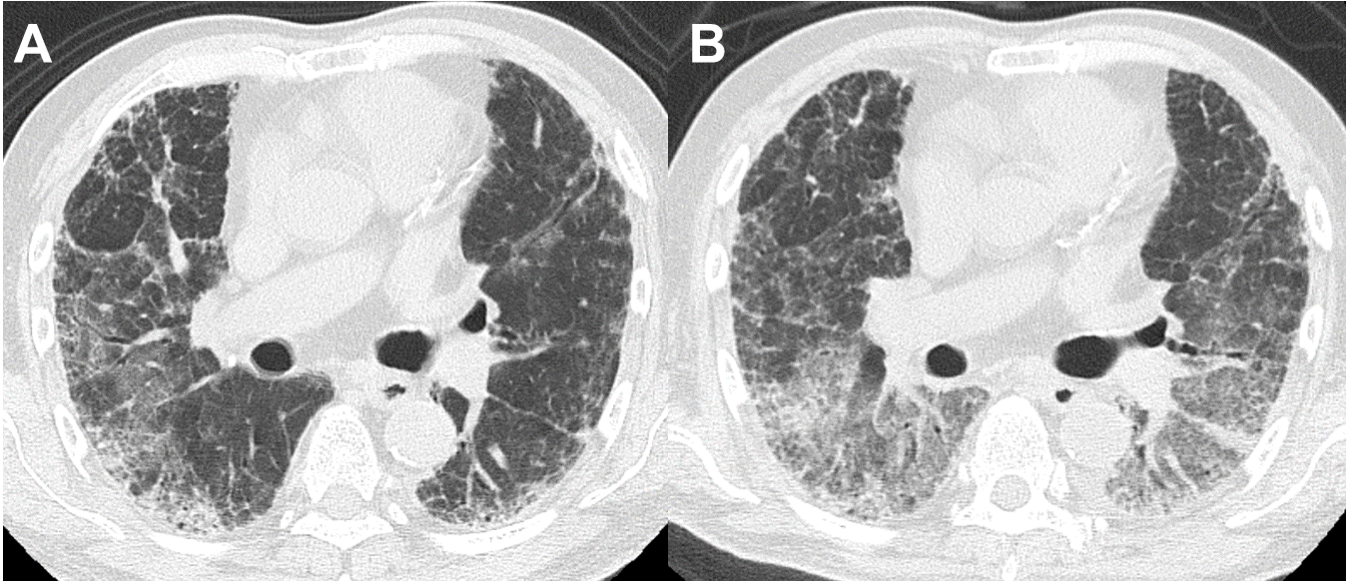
A patient in their 60s with chronic hypersensitivity pneumonia. (A) HRCT before the BAL procedure showed reticular shadows with bronchodilation in the peripheral lesion. (B) Two days after the BAL procedure, which was performed from B3b on the right lung, ground-glass opacities extended to both lungs.
